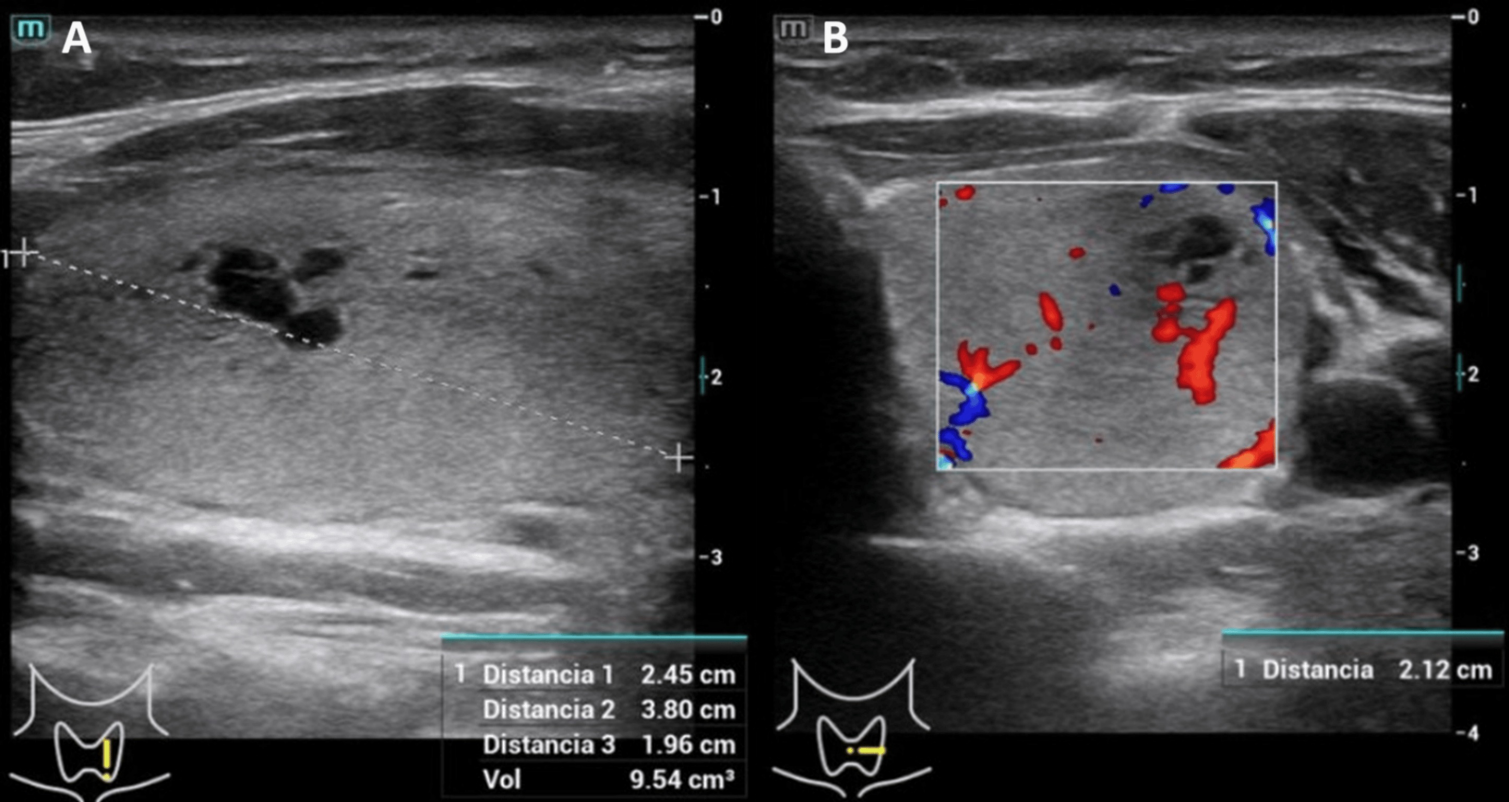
Thyroid ultrasound Thyroid ultrasound. (A) Compensatory hypertrophy of the left lobe (9.5 g) containing a 21 x 36 mm dominant nodule with central cystic degeneration. (B) Color Doppler showing discrete vascularity (TI-RADS 4A). TI-RADS: Thyroid Imaging Reporting and Data System
